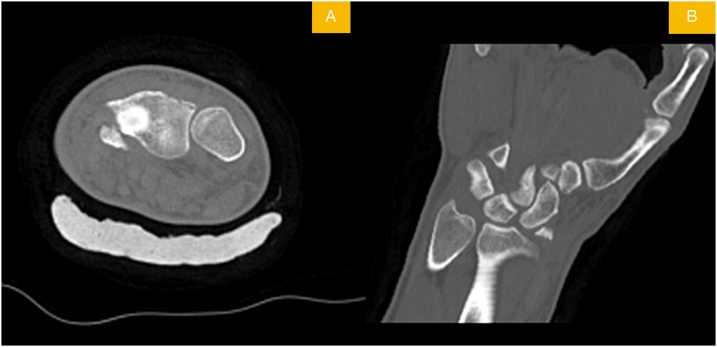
Axial (A) and coronal (B) CT scan views of displaced radial styloid fracture.
